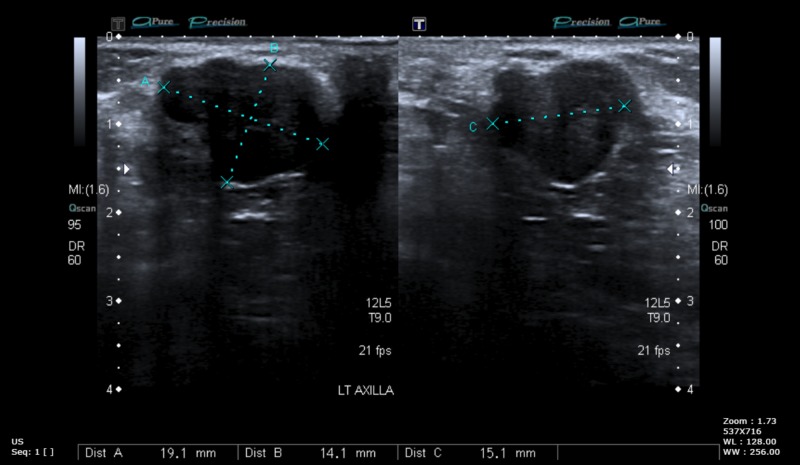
USG: Hypoechoic lesion USG: ultrasonography
